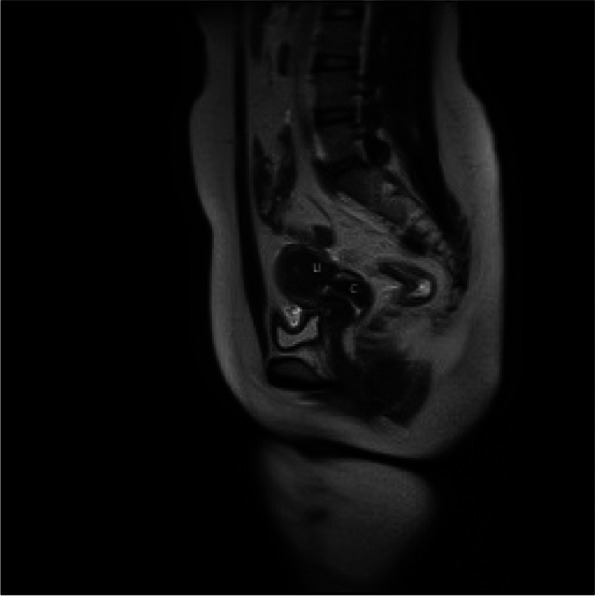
MRI examination three months after surgery showed a normal uterus (U) and cervix (C) with the myometrial continuity of the isthmus
